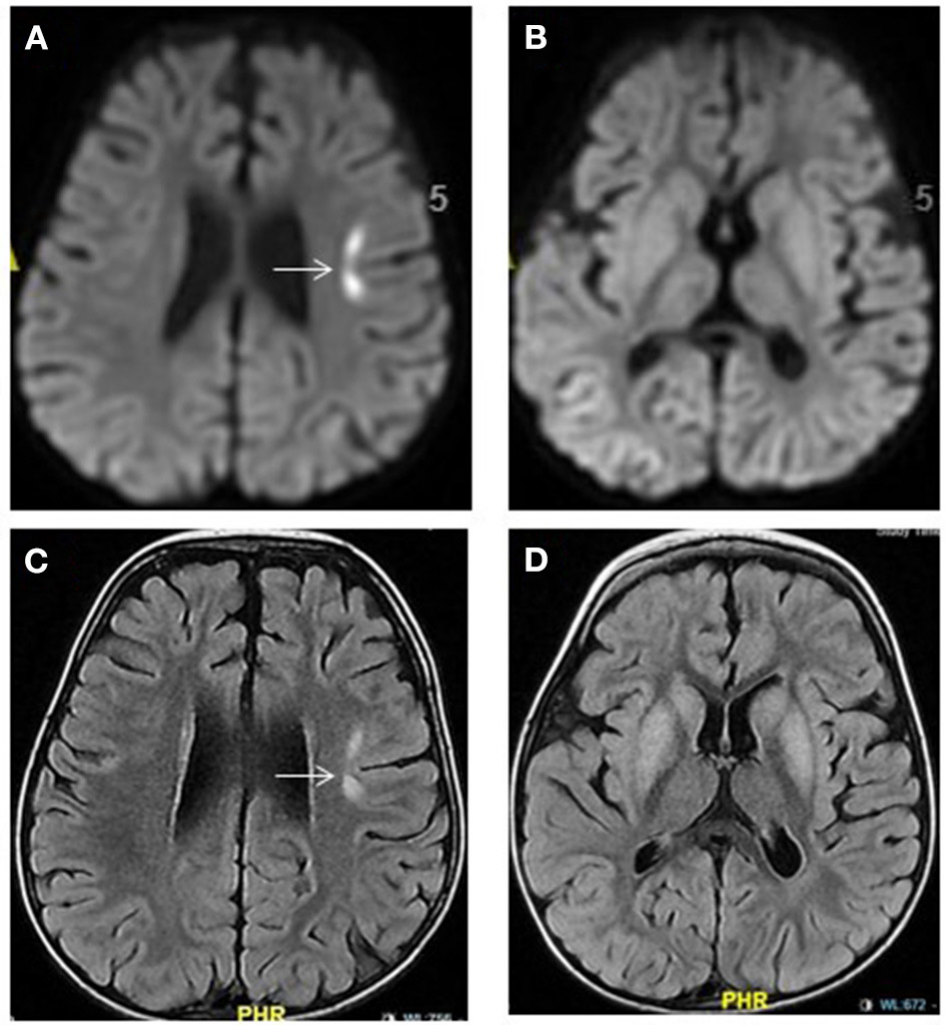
Propionic acidemia. Brain MRI: Axial diffusion-weighted imaging (A,B) and FLAIR images (C,D) show hyperintensities in the bilateral basal ganglia and in the left frontal cortical-subcortical focal area (arrow). The latter showing diffusion restriction (A) representing acute injury. No diffusion restriction within the basal ganglia seen (B).
